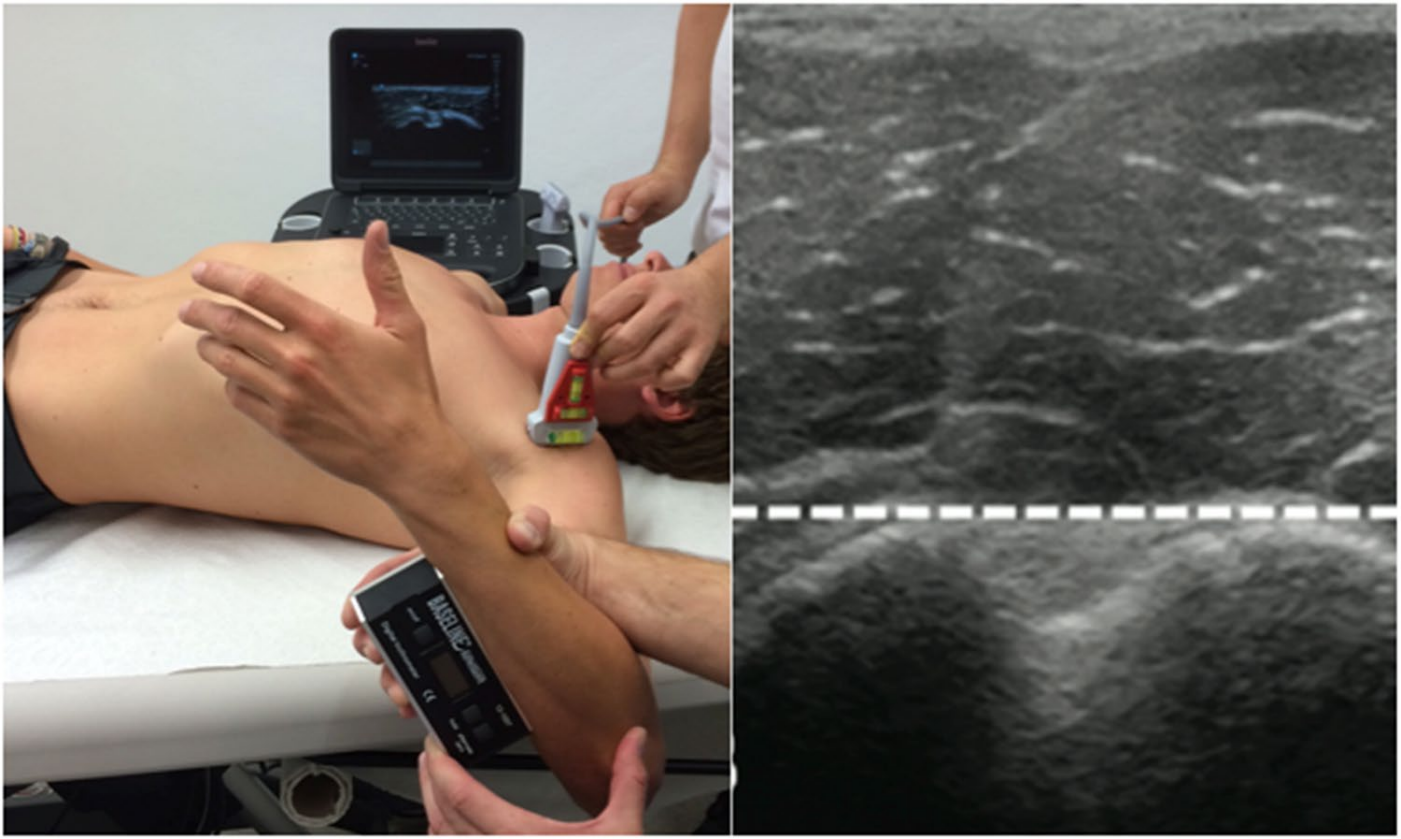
Left: Technique of indirect sonographic assessment of humeral torsion. Right: Ultrasonographic image of the proximal humerus with the apices of the humeral tubercles aligned horizontally
